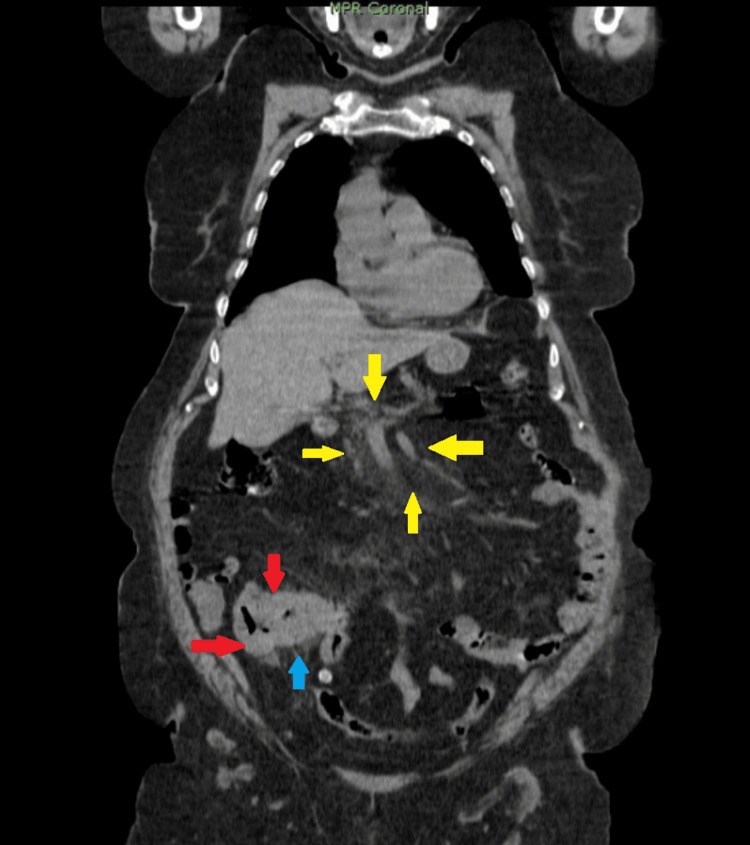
Coronal contrast-enhanced CT reconstruction showing the relationship between the cecal lesion and mesenteric panniculitis Coronal contrast-enhanced CT reconstruction showing irregular cecal wall thickening suspicious for an underlying malignant lesion, adjacent fat infiltration, and associated mesenteric panniculitis. Red arrow indicates the suspicious cecal wall thickening, blue arrow indicates adjacent fat infiltration likely related to the cecal lesion, and yellow arrow indicates mesenteric inflammatory fat changes consistent with mesenteric panniculitis. CT: computed tomography
